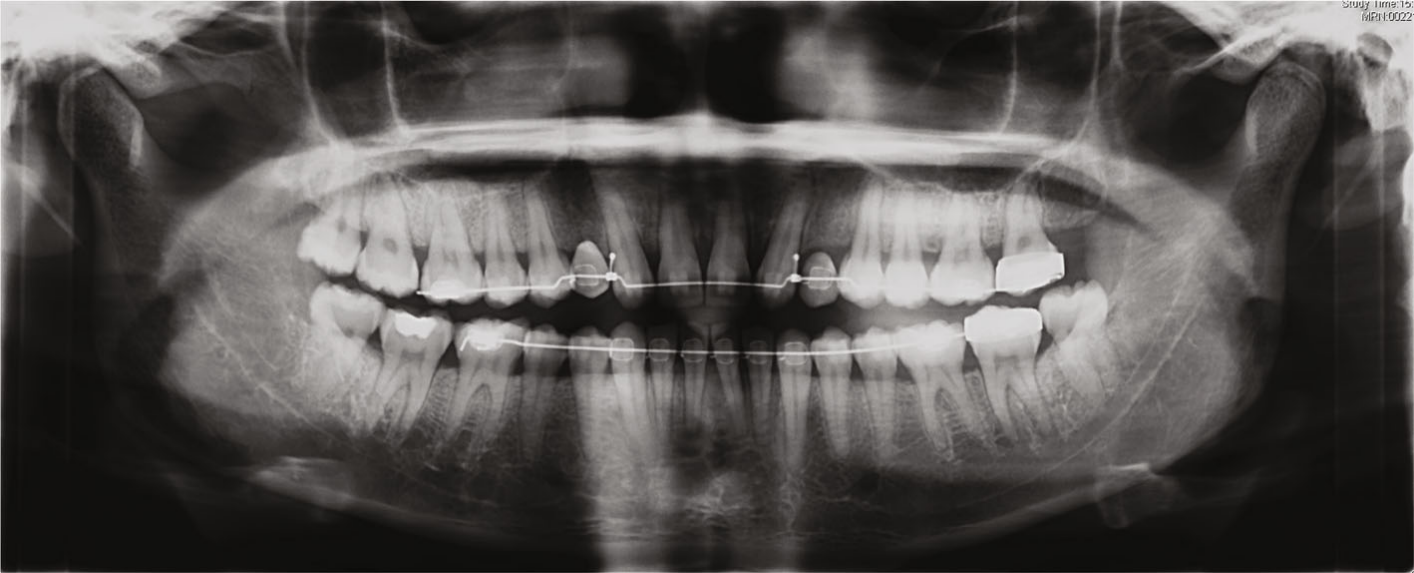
Panoramic radiography at the end of the orthodontic treatment.
